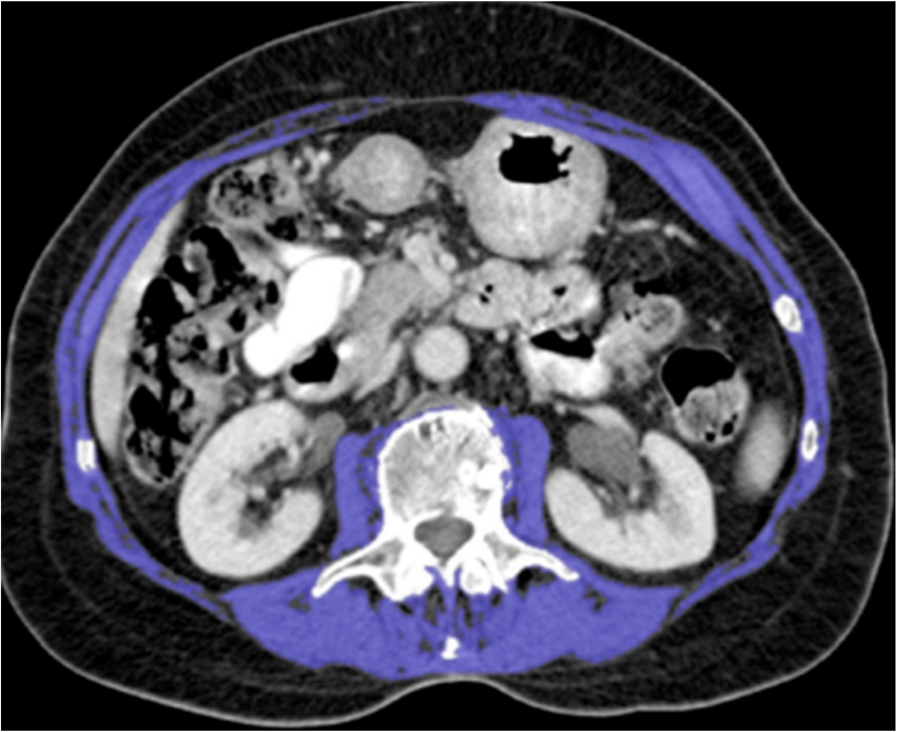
Example of computed tomography (CT) image analysis methods on Osirix version 5.6.1 open source software
